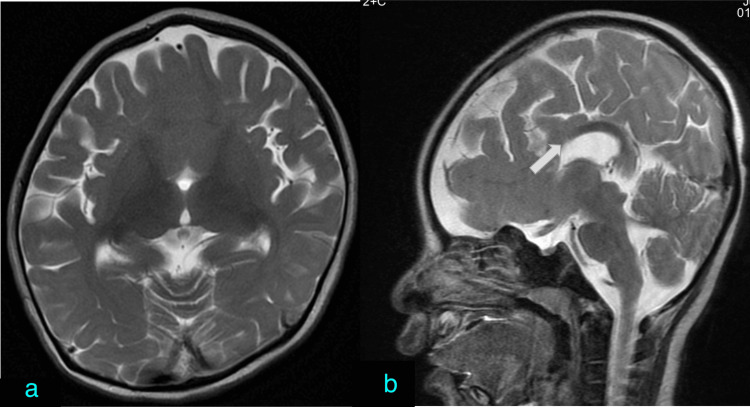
Corpus callosum agenesis with holoprosencephaly. (a) Axial T2-weighted imaging showing fusion of the anterior bilateral frontal lobes with the absence of anterior interhemispheric fissure. (b) Sagittal T2-weighted imaging showing the absence of anterior corpus callosum.
